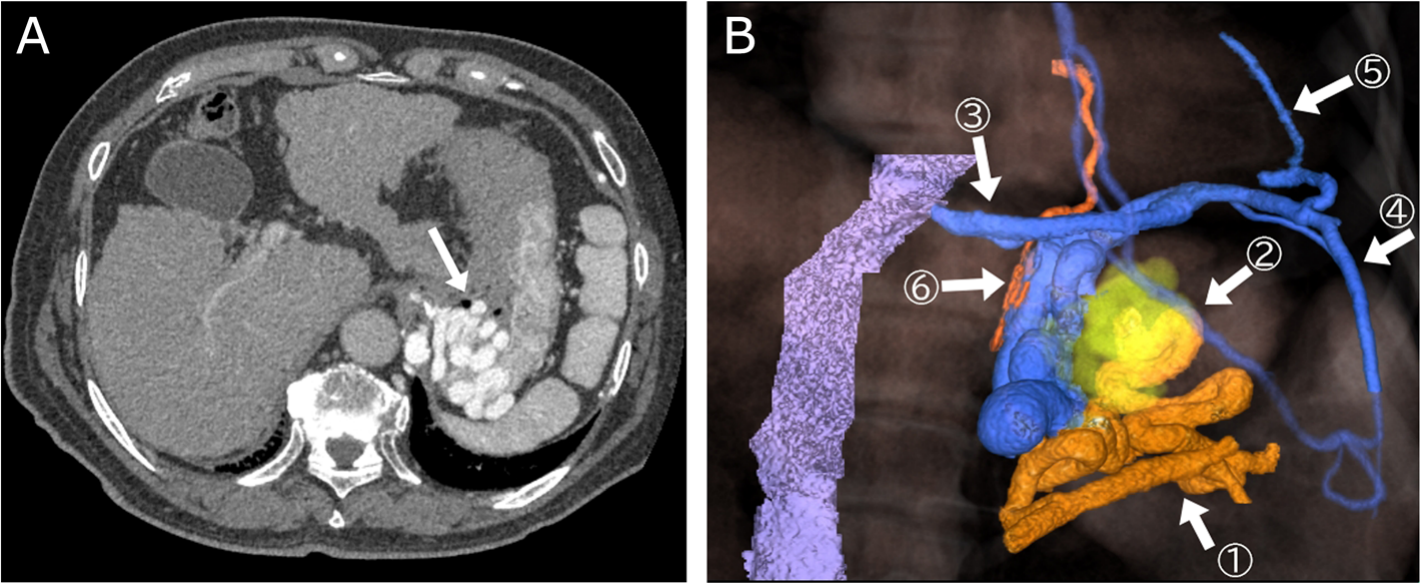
a Representative computed tomography during arterial portography image showing gastric varices (white arrow) before treatment. b Three-dimensional volume rendered images of gastric varices reconstructed from computed tomography during arterial portography. The main feeding vessels of gastric varices are the posterior and short gastric vein (①). The varices (②) drained mainly into the left inferior phrenic vein (③), the gastrorenal shunt was absent. The left internal thoracic vein (④), pericardial phrenic vein (⑤), and left inferior pulmonary vein (⑥, which is from the outflow shunt) were other drainage veins. Mild stenosis is observed between the inferior vena cava and left inferior phrenic vein.
